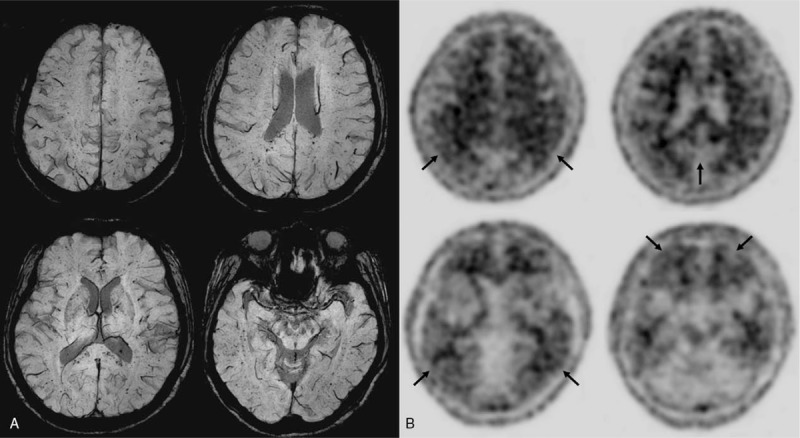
(A) Brain MRI (SWI sequence). There are innumerable cerebral microbleeds at the bilateral frontal, parietal, temporal, and occipital lobes. (B) The ^18^F-florbetaben PET images. Visual assessment of the four main target regions based on RCTU and BAPL scoring judged as positive for β-amyloid plaques (BAPL 3, parietal = RCTU 3; posterior cingulate/precuneus = RCTU 1; lateral temporal = RCTU 1; frontal = RCTU 1). BAPL = brain β-amyloid plaque load, MRI = magnetic resonance imaging, PET = positron emission tomography, RCTU = regional cortical tracer uptake, SWI = susceptibility weighted imaging.
